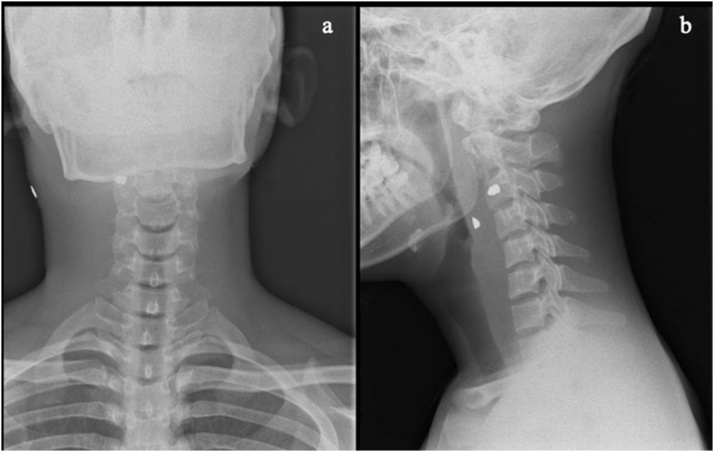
Cervical spine X-Ray. A: Anteroposterior view; B: Lateral view showed the bullet at the level of the third cervical vertebra.
